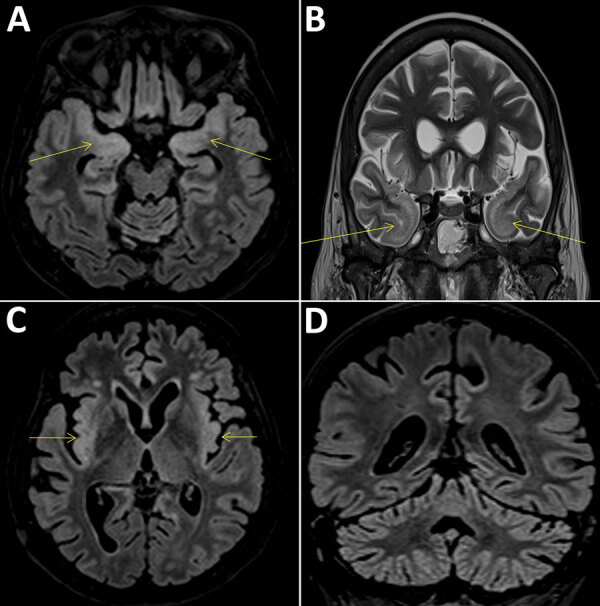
Cerebral magnetic resonance imaging scans compatible with the diagnosis of encephalitis in a 58-year-old woman, France. Fluid-attenuated inversion recovery (FLAIR) and T2 hypersignals in limbic system structures, including both amygdalae (A, arrows), temporal poles (B, arrows), and insular cortex (C), associated with FLAIR hyperintensities of the cerebellar cortex (D).
